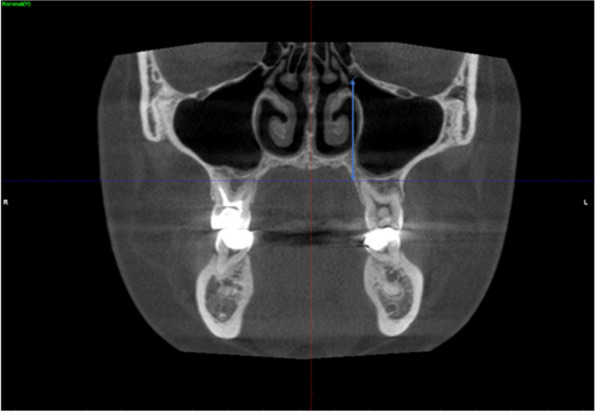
Coronal CBCT images showing the ostium height
